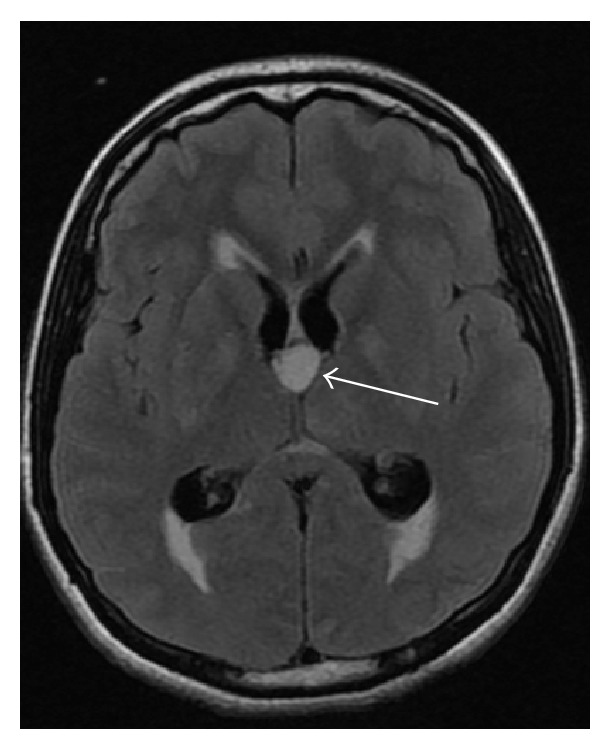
MRI brain FLAIR image axial view showing round shaped mass in the third ventricle, with enlarged both lateral ventricles shown by white arrows.
